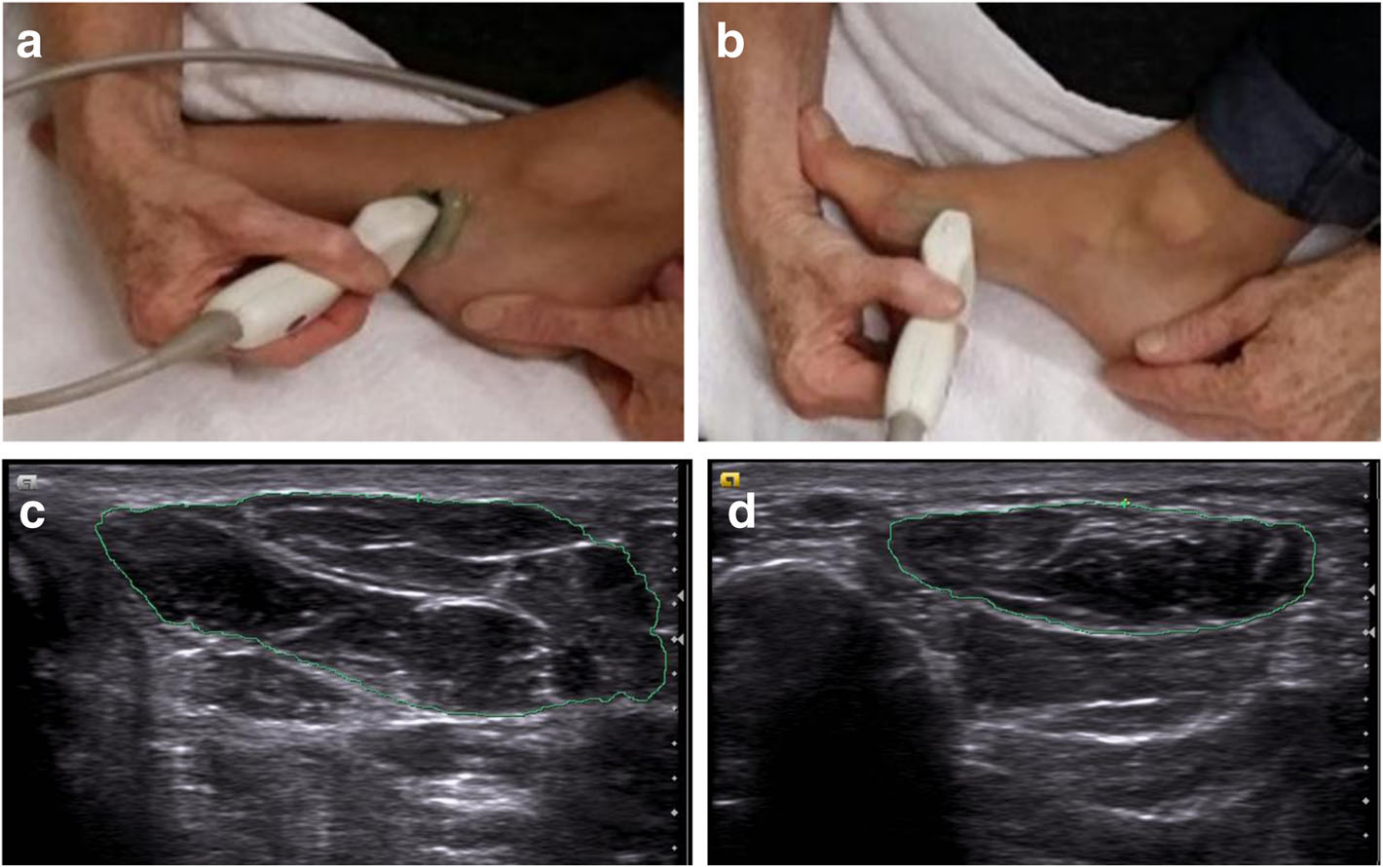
Ultrasound transducer placement, scanned image and outlined circumference. a Transducer placement to scan the AbH muscle, b Transducer placement to scan the FHBM muscle, c Ultrasound image of the cross-sectional area of AbH outlined, d Ultrasound image of the cross-sectional area of FHBM outlined
